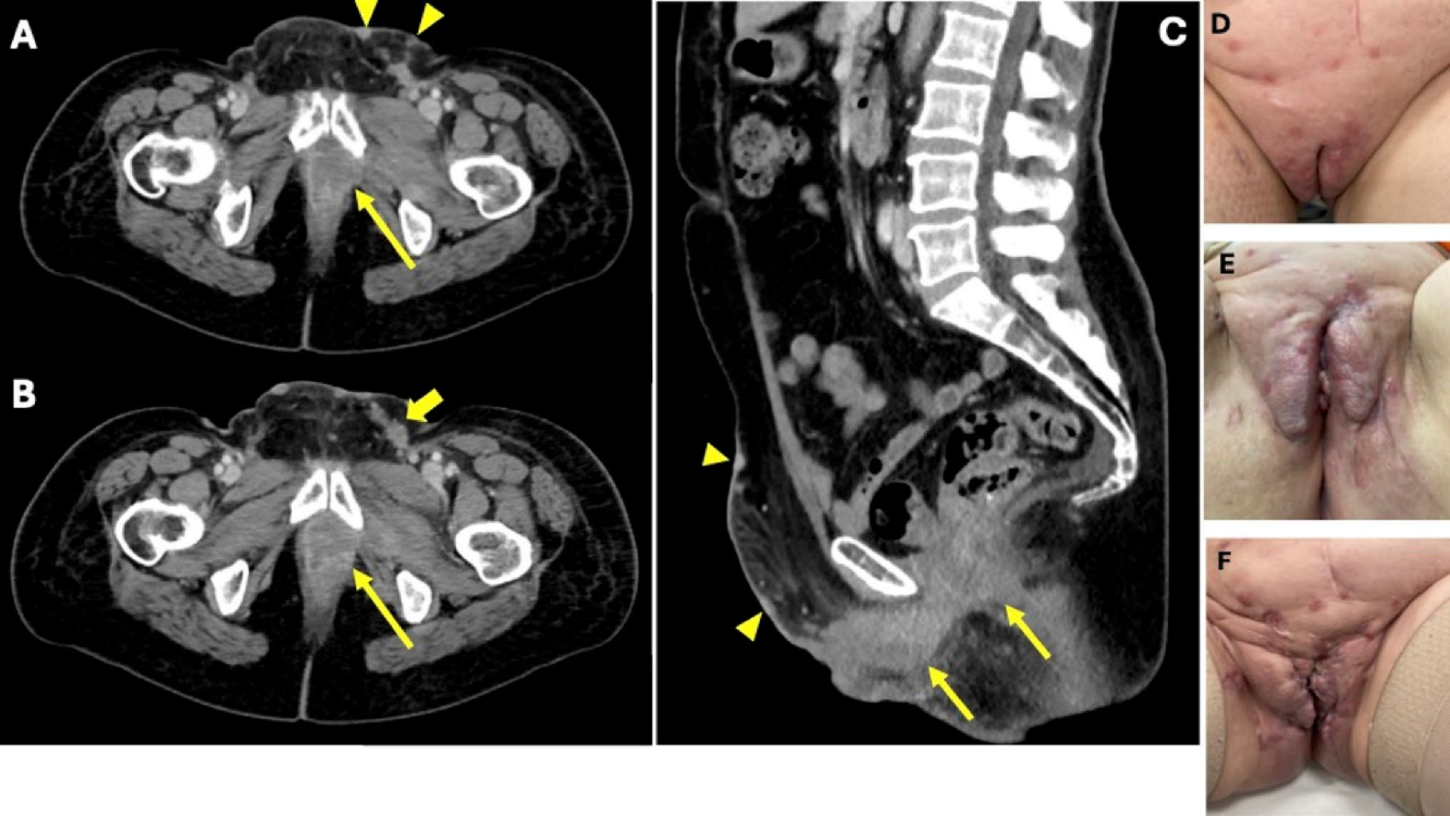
Representative CT findings of patient 4, of the abdomen. A and B: Axial CT-scans at her 1st recurrence before the start of systemic therapy. C: Sagittal CT-scan at her last presentation after 3 cycles of Carboplatin/Paclitaxel/Pembrolizumab. Tumor behind the symphysis (yellow arrows). Skin metastases (yellow arrow heads). Inguinal lymph node metastasis (thick yellow arrow). D, E, F: Photographs of the local progression under systemic therapy. D: before the start, E: after 1 cycle of therapy, F: after 3 cycles of therapy
